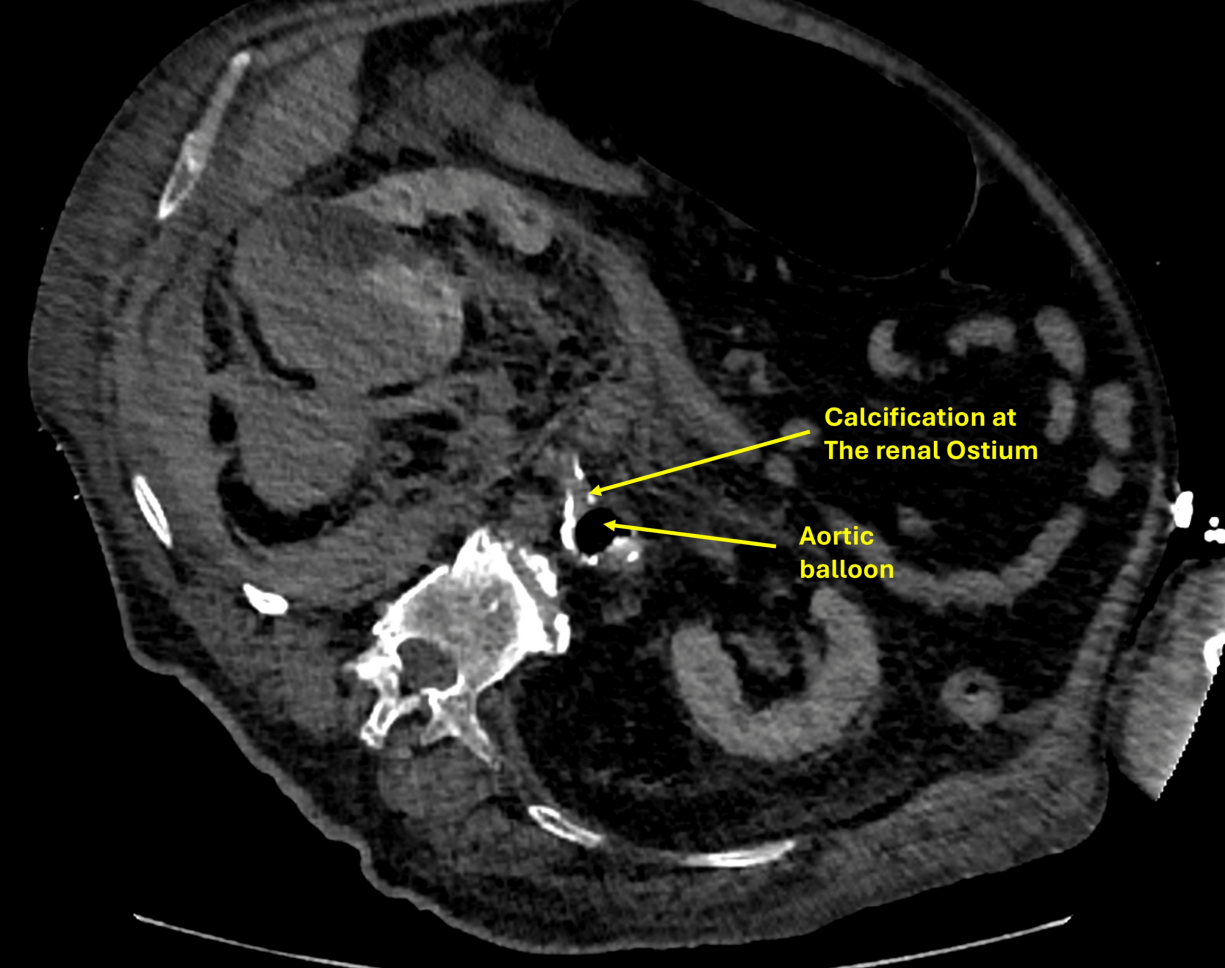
CT of the abdomen showing a calcified renal artery ostium and an intra-aortic balloon pump in place.
